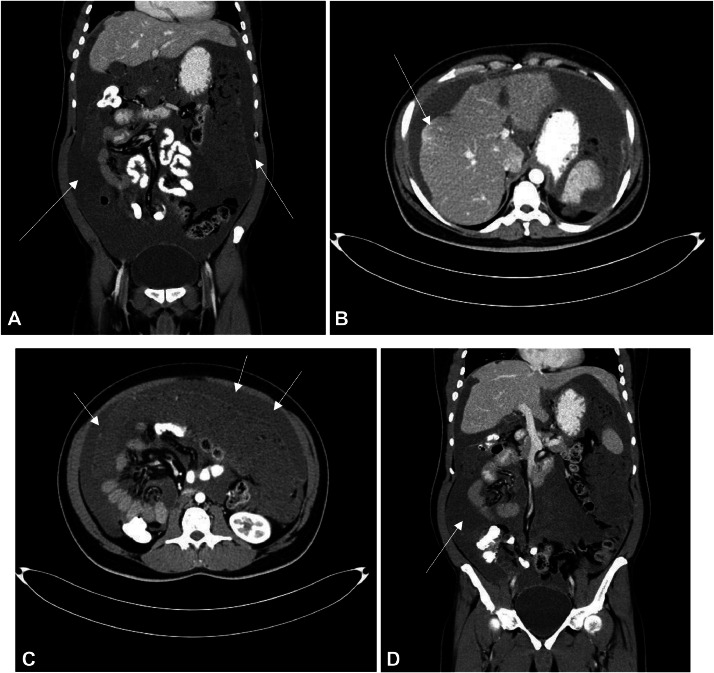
Abdominopelvic CT scan with contrast denoting: (A) Extensive ascites on coronal section (B) axial section at the level of the liver showing small indentation of the hepatic surface by the fluid collection impressive of mucinous content (C) Axial section postcontrast at the level of kidneys showed extensive omental thickening. (D) Coronal section postcontrast demonstrating soft tissue thickening seen related to the cecum, otherwise, the appendix is not well-delineated.
